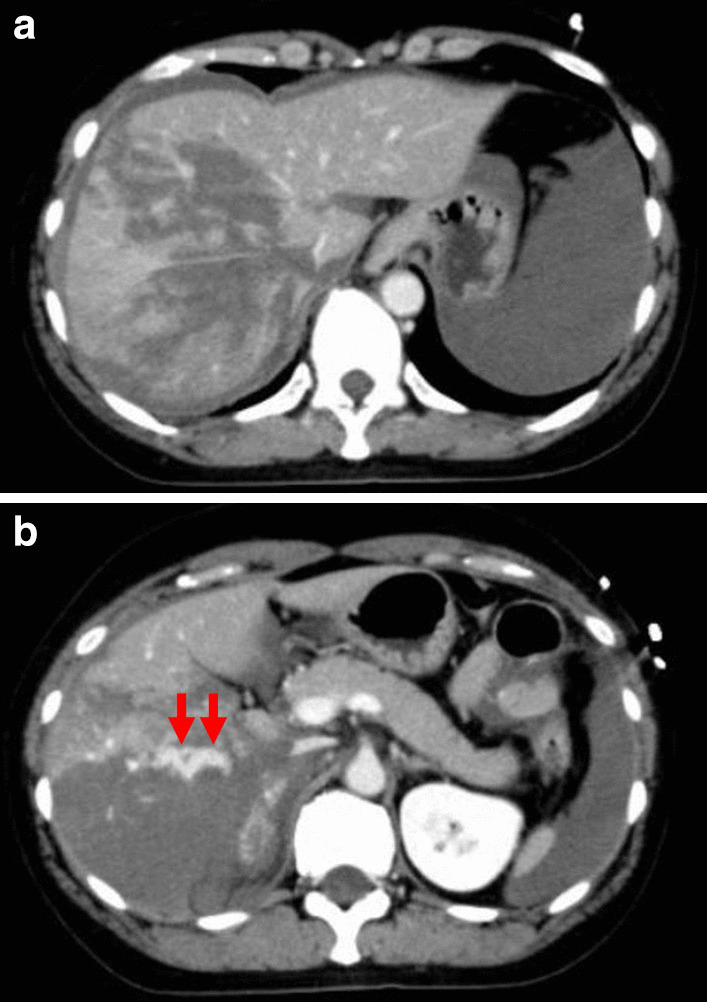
Abdominal CT scan taken at the local hospital prior to transportation to the University Hospital. a Irregular and mottled devascularized area occupied the whole right lobe of the liver. b Contrast extravasation from right hepatic artery (arrow) and hemoperitoneum
